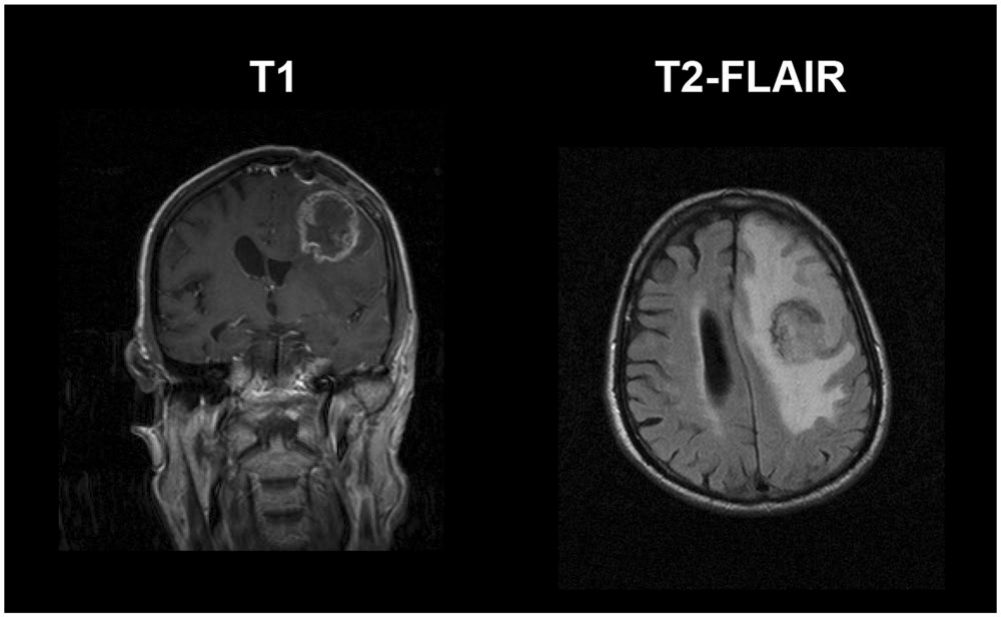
Post-biopsy Magnetic Resonance (MR) images from a 76-years-old male GB patient. On the left, a coronal T1-MR image, where the highly necrotic ring-shaped lesion can be seen frontotemporally in the left hemisphere, near motor area. Notice the mark of biopsy cavity formed by the trepanation of the skull. On the right, an axial T2-FLAIR MR image is shown, where the bright peritumoral infiltrative edema is remarkably extended causing space-occupation of the left hemisphere and internal pressing of the lateral ventricle.
